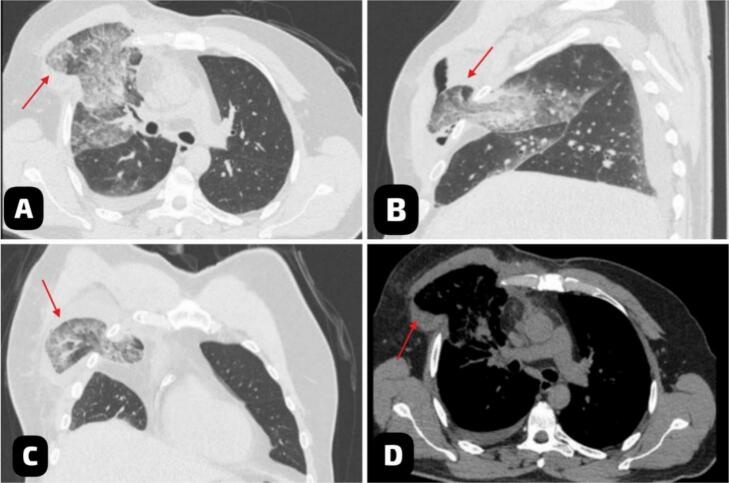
Multiplanar chest CT scan without IV contrast in lung windows (A, B, C) and mediastinal window (D) showed intercostal herniation of right lung parenchyma (red arrow). (For interpretation of the references to colour in this figure legend, the reader is referred to the web version of this article.)
